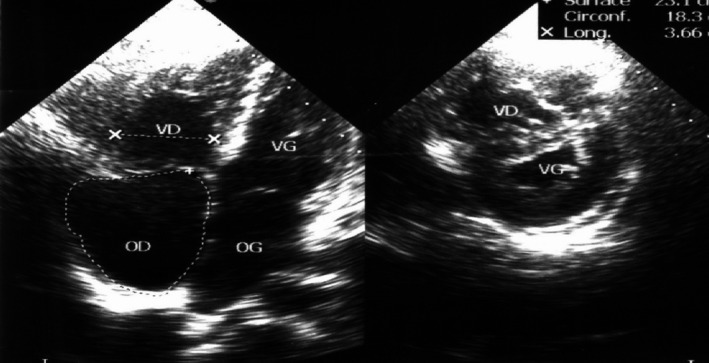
Echocardiogram of case number 1 shows markedly dilated right cavities with mass effect on the left cavities. The pulmonary systolic pressure was estimated at 85 mmHg.
